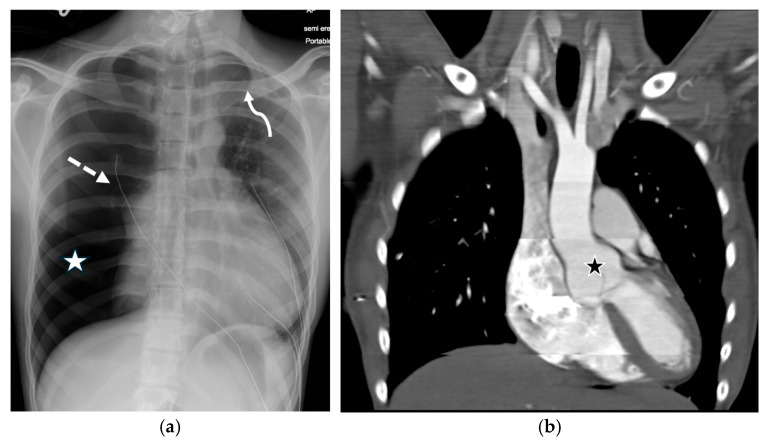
17-year-old male with Ehlers–Danlos syndrome presents with chest pain. Frontal chest radiograph (a) shows large right-sided pneumothorax (white star) under tension (as manifested by leftward mediastinal shift and inferior diaphragmatic shift), small left-sided pneumothorax (curved white arrow), and right apical bleb (dashed white arrow). Coronal contrast-enhanced CT image in soft tissue window (b) demonstrates dilated aortic root (black star).
